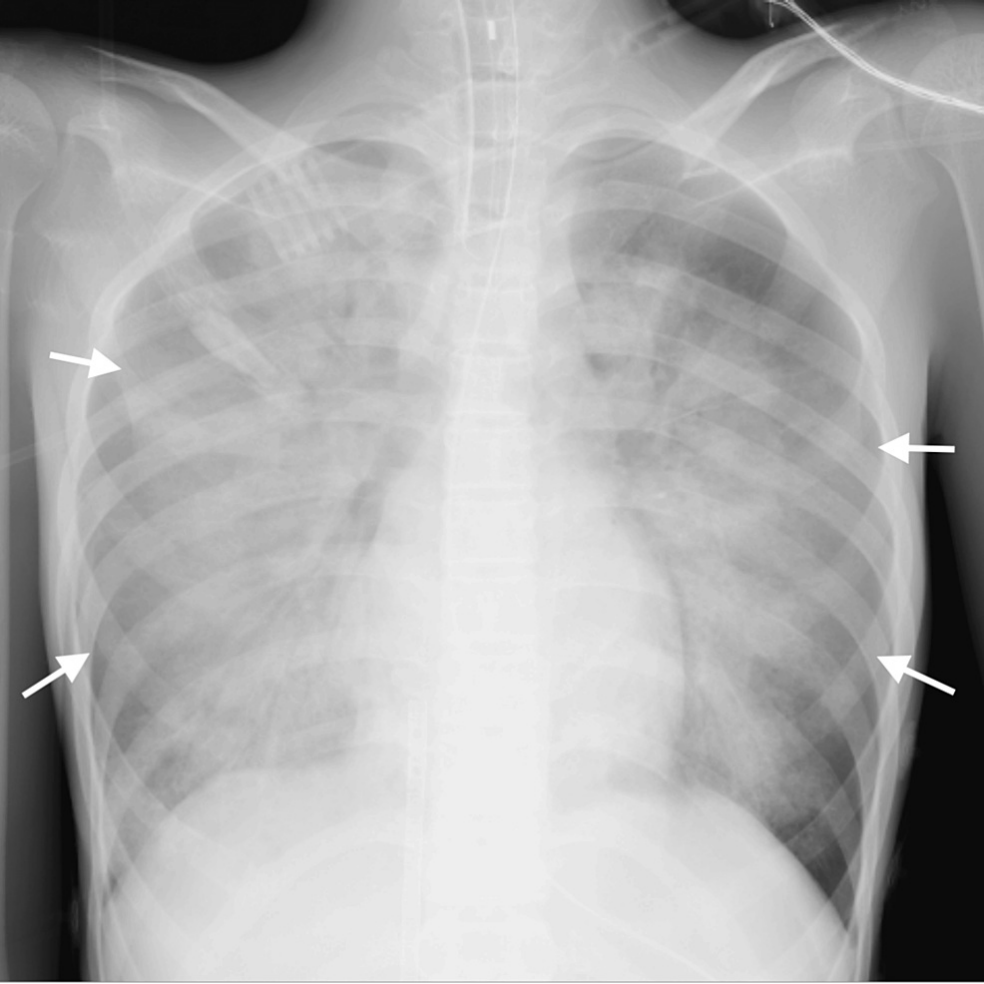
Chest radiography showed extensive bilateral pulmonary edema on admission
